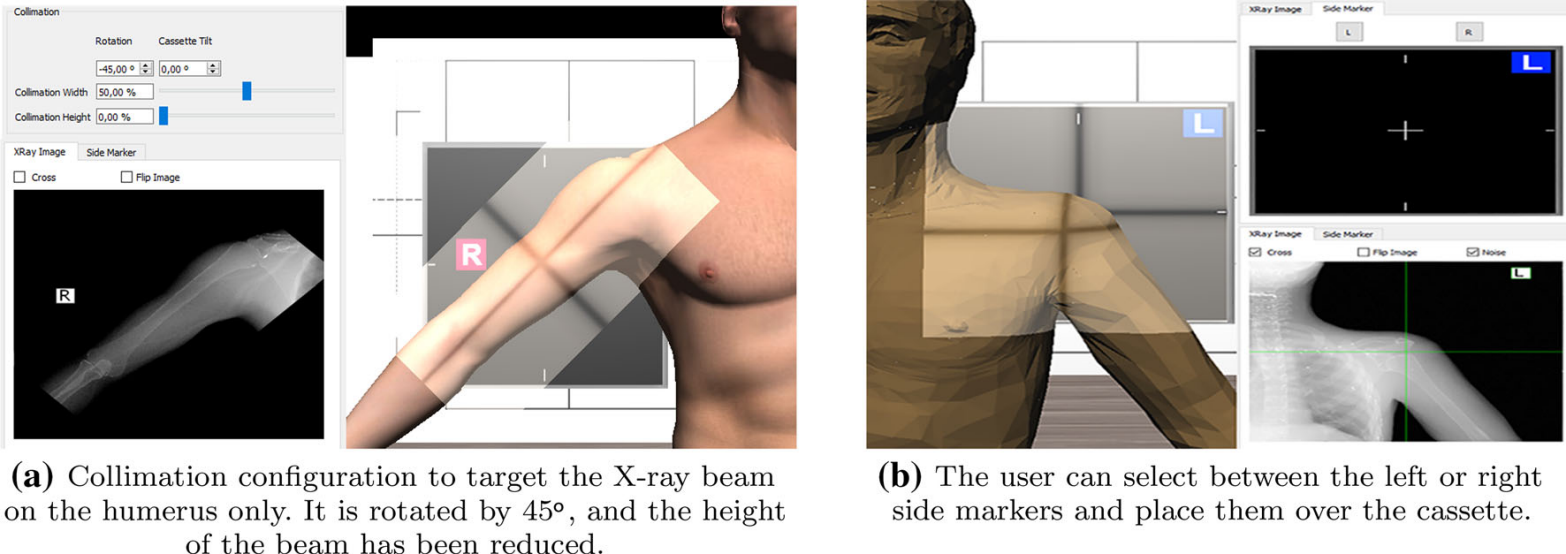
Users can move and configure the cassette to get an X-ray image
